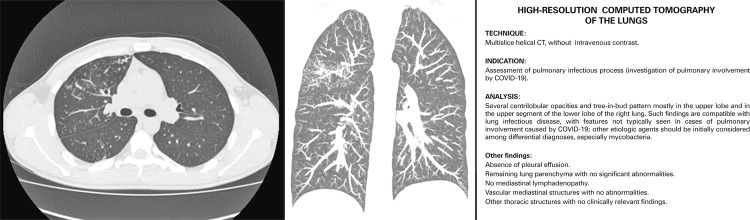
Computed tomography images of a case with initial clinical suspicion of COVID-19 and its respective report, with tomographic findings suggestive of infectious process, but with characteristics that are not usual in COVID-19. After performing ancillary investigations, the diagnosis was pulmonary tuberculosis
